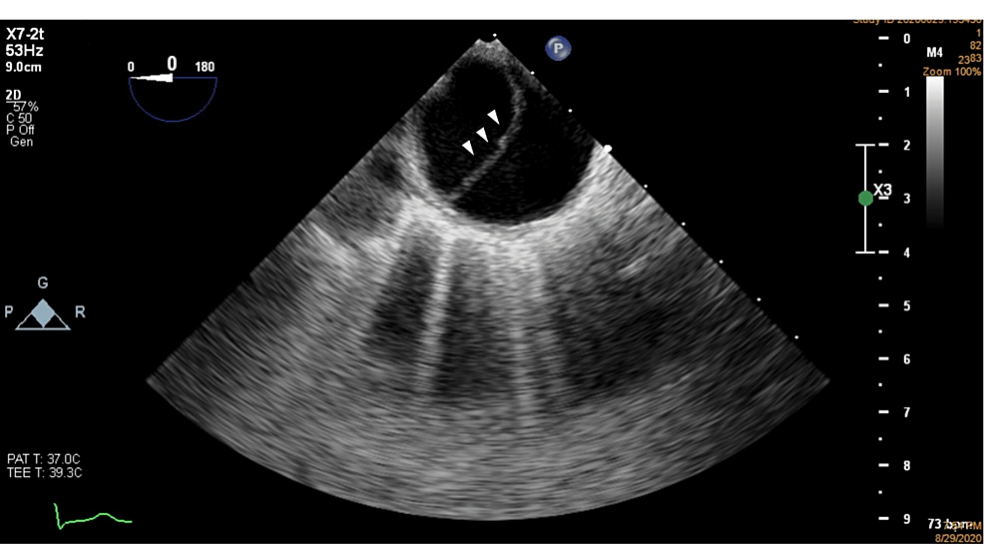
Aortic evaluation by TEE showing flap image (arrowhead) in the thoracic aorta distal to the emergence of the left subclavian artery. TEE: transesophageal echocardiography
